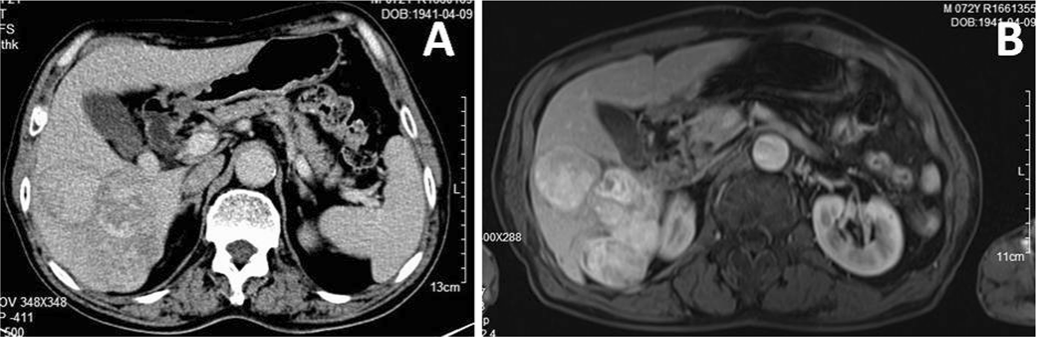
Imaging findings of case 1. (A), (B) Computed tomography and magnetic resonance imaging showing a mass in the right liver lobe.
